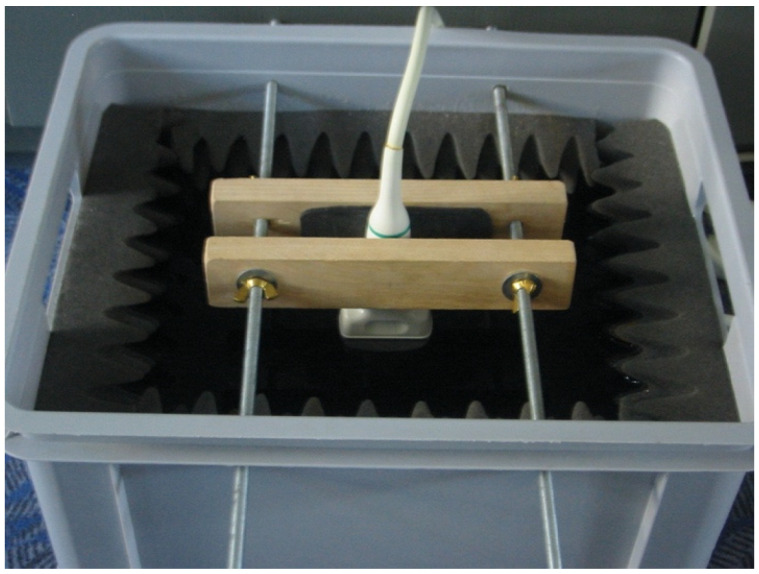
Probe placement in a standardized position. The bucket holds distilled water and is insulated to prevent ultrasonic reverberations. The metal frame is positioned inside. Figure 1, Figure 2 and Figure 3 were provided with kind permission from H. Dudwiesus.
